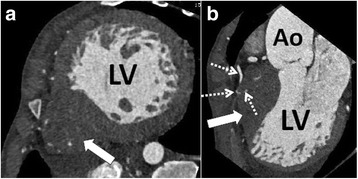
Cardiac CT of a 1 month old boy showing univentricular left ventricle (LV) and rudimentary right ventricle (a and b; arrows). Coronary artery fistula from right coronary artery draining into the rudimentary right ventricle was found (b; dashed arrows)
